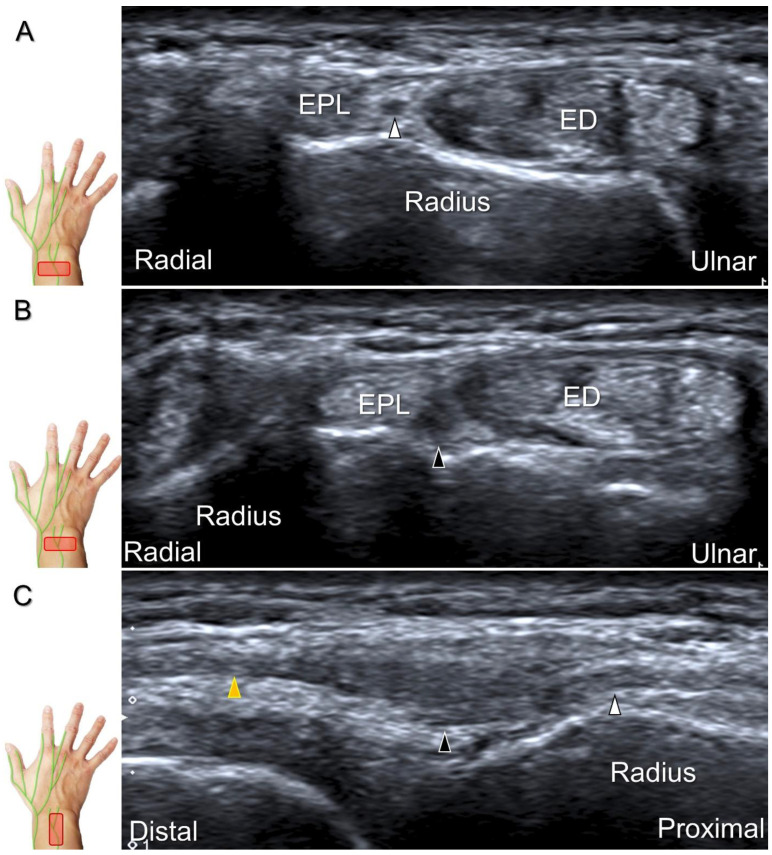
Sonographic imaging (short-axis view) of the dorsal interosseus nerve from the normal (A) to the swollen (B) segment proximal to the entrapment site. Normal, swollen, and compressed segments of the nerve are seen in long-axis view (C). White arrowhead: normal segment; black arrowhead: swollen segment; yellow arrowhead: entrapped segment; EPL: extensor pollicis longus tendon; ED: extensor digitorum tendon.
